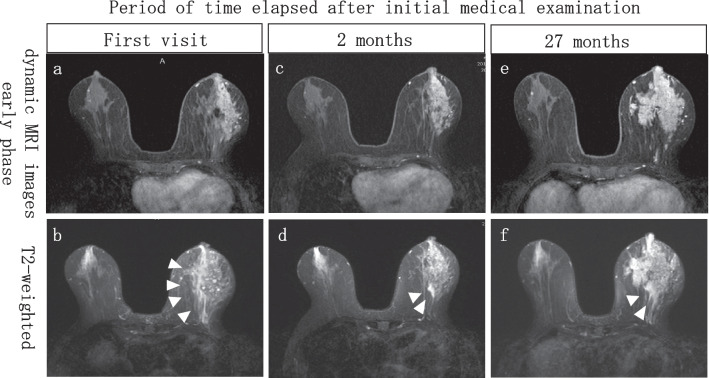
Changes in MRI findings. a, b At initial diagnosis, c, d 2 months after initial diagnosis, e, f at DCIS diagnosis. The high-signal intensity of the dorsal part of the mammary gland in the fat-suppressed T2-weighted image is shown with arrowheads. MRI, magnetic resonance imaging; DCIS, ductal carcinoma in situ
